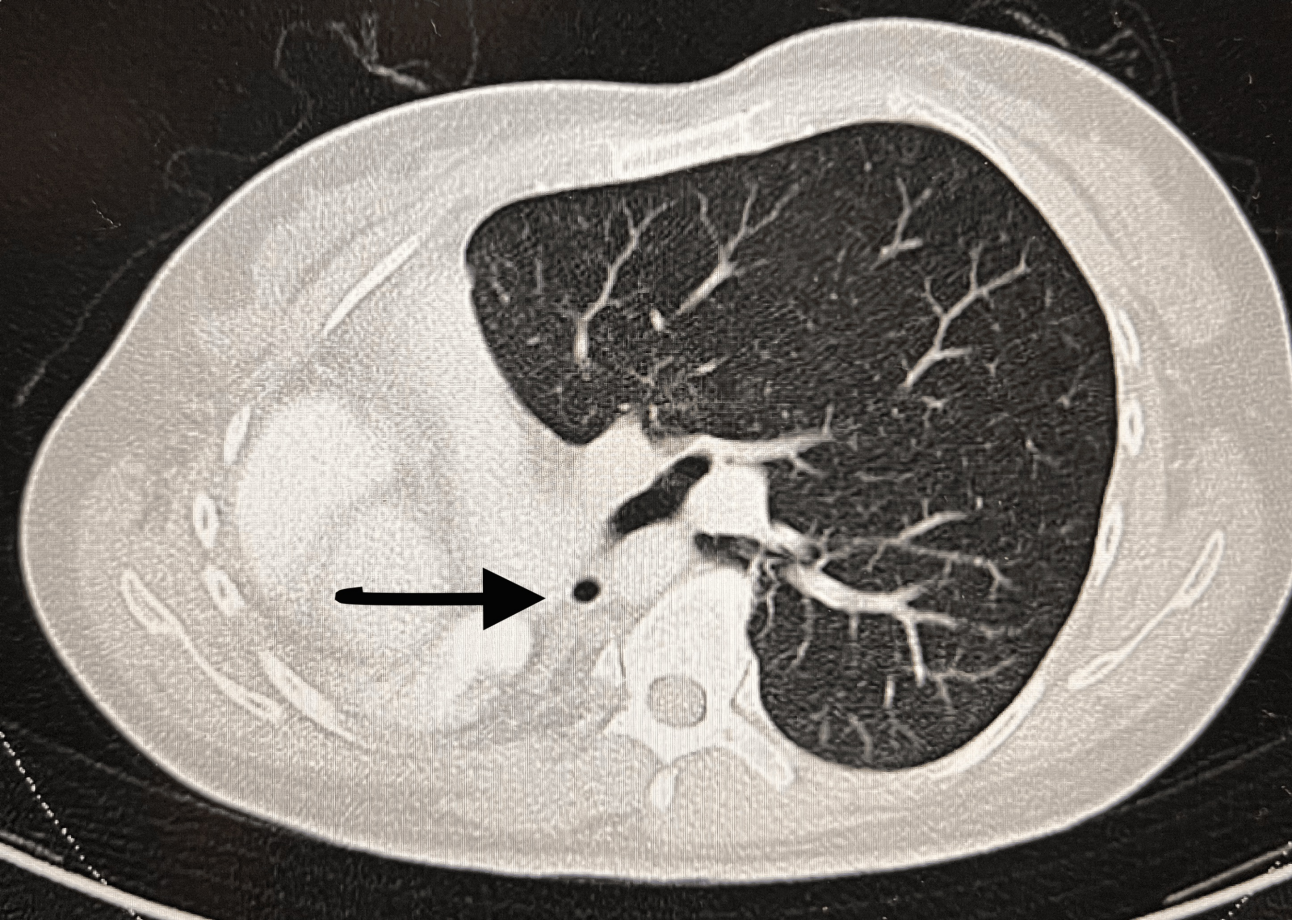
CT chest showing the stump of the right bronchus and absence of the right lung.
